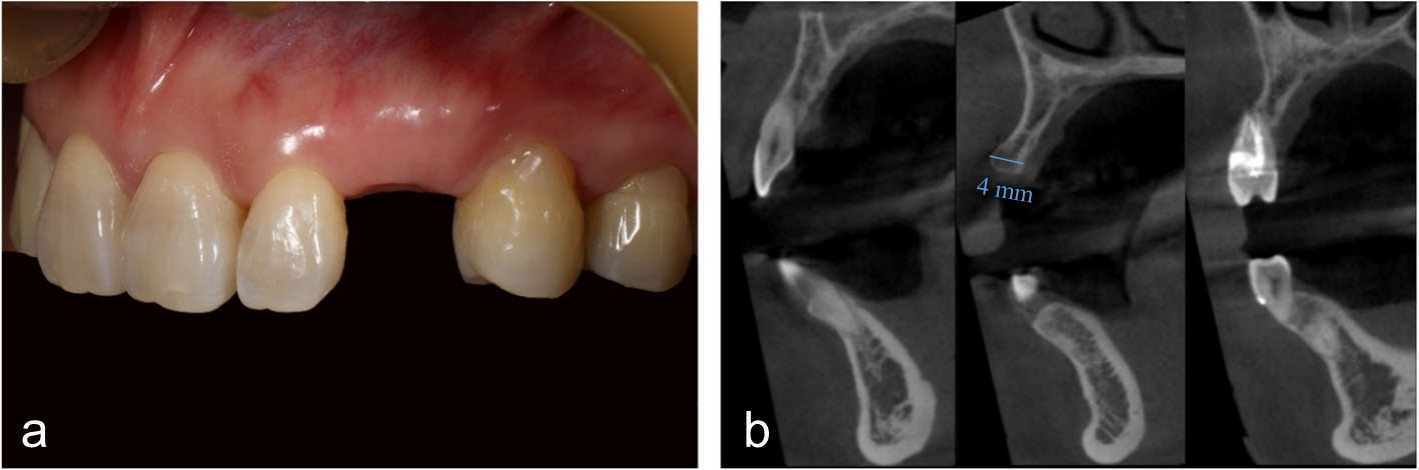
Preoperative examination. a Intraoral photograph; b severe horizontal bone defect revealed on CBCT
